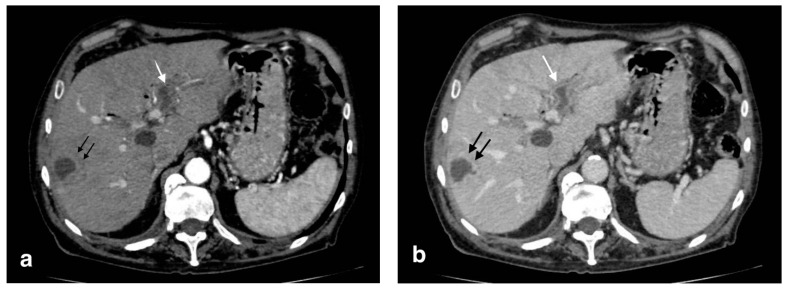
Liver abscess with pylephlebitis: (a) CT arterial phase; (b) CT portal phase. In the right lobe, V segment, a large hypodense collection with hypoattenuating halo due to intrahepatic abscess (black arrows, (a,b)) with the presence of a hypodensity within the left portal vein lumen due to thrombosis and hyperdensity of portal walls indicative of pylephlebitis better defined in CT portal phase (b) (white arrow).
